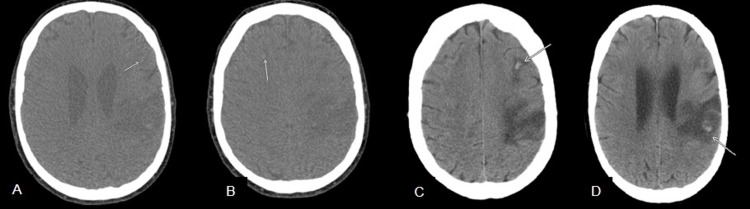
A-D. Initial CT head obtained at tertiary care facility displaying multiple foci hemorrhages within bilateral frontal and right temporal lobe. Arrows indicate multiple foci of hemorrhage.
